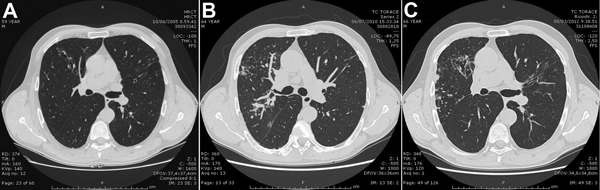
High-resolution computed tomographic chest images of a man with prolonged pulmonary disease caused by *Mycobacterium marseillense,* Italy. A) October 6, 2005. Bilateral bronchiectasis, mainly in the middle lobe and lingula, associated with multiple nodules and middle lobe consolidation. B) June 7, 2010. Increased micronodular opacities, mainly in the right middle and lower lobes, and a worsening of the bronchiectasis. C) August 3, 2013. Persistence of nodular component, cavitation, and wider bronchiectasis with bronchial wall thickening.
